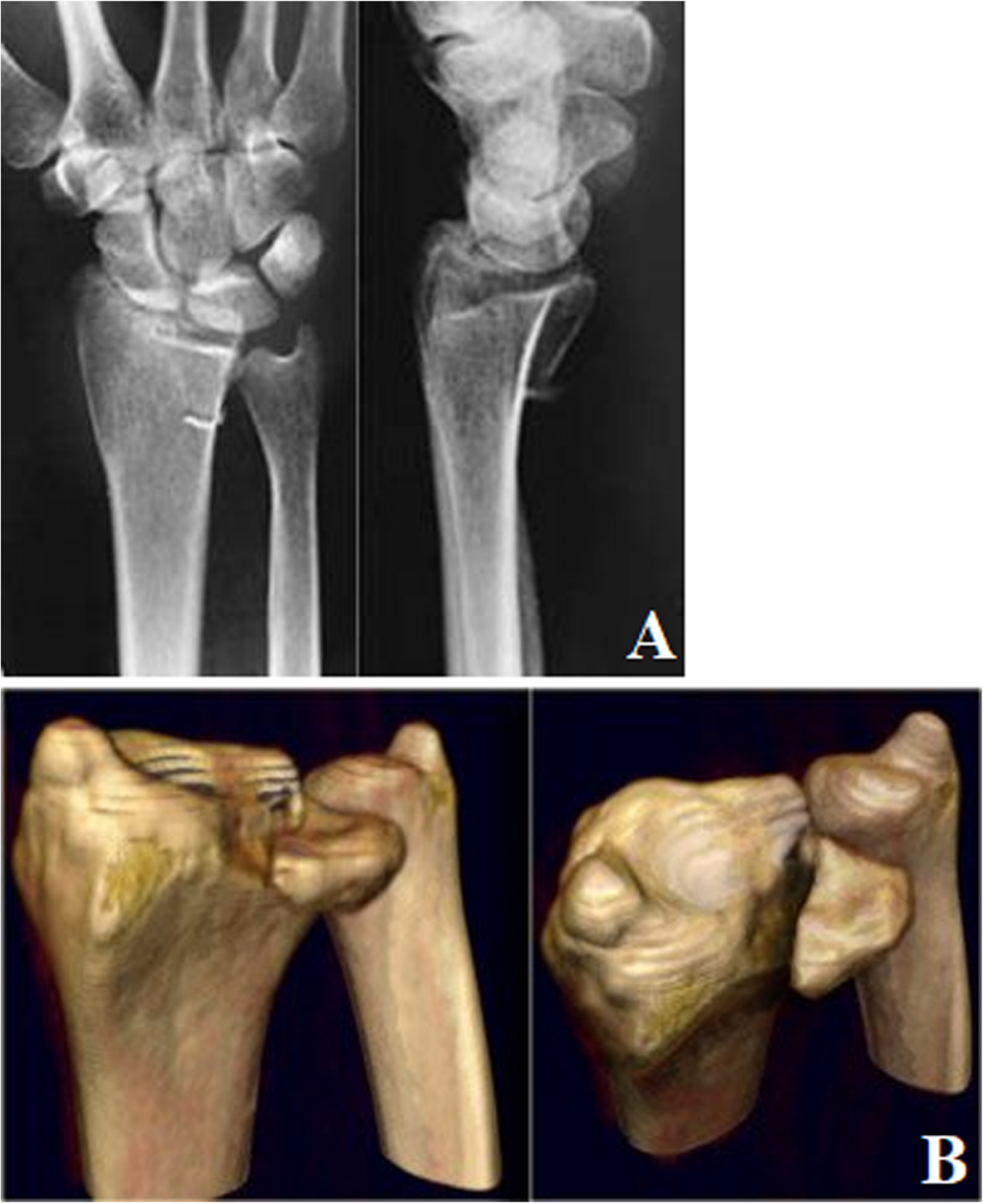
PA X-ray (a) and CT scan (b) of volar single-column DRDPF
